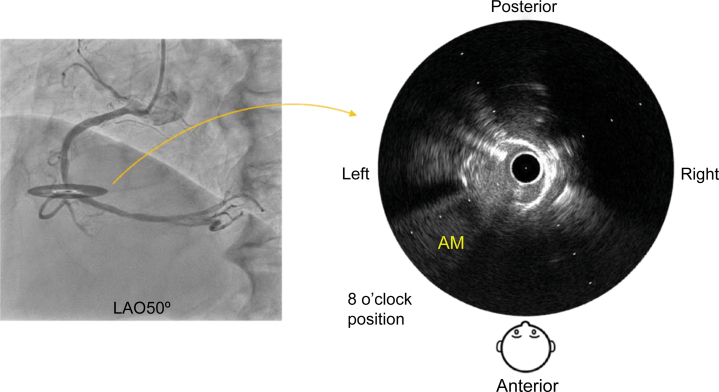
Bifurcation After “Fusion” On the IVUS image, the opening of the acute marginal branch is in approximately the 8 o'clock position, and on the angiographic image, it can be seen to open from the left anterior direction. AM = acute marginal; IVUS = intravascular ultrasound.
